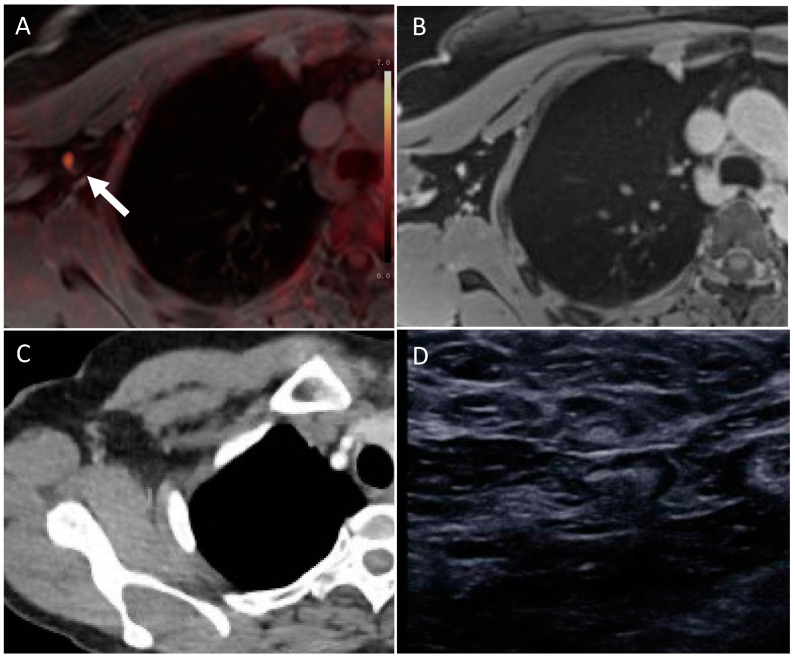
Lymph node metastases in axillary level I on the left side, correctly identified by ^18^F-FDG PET/MRI ((A), see white arrow), but missed by MRI (B), CT (C) and axillary sonography (D).
